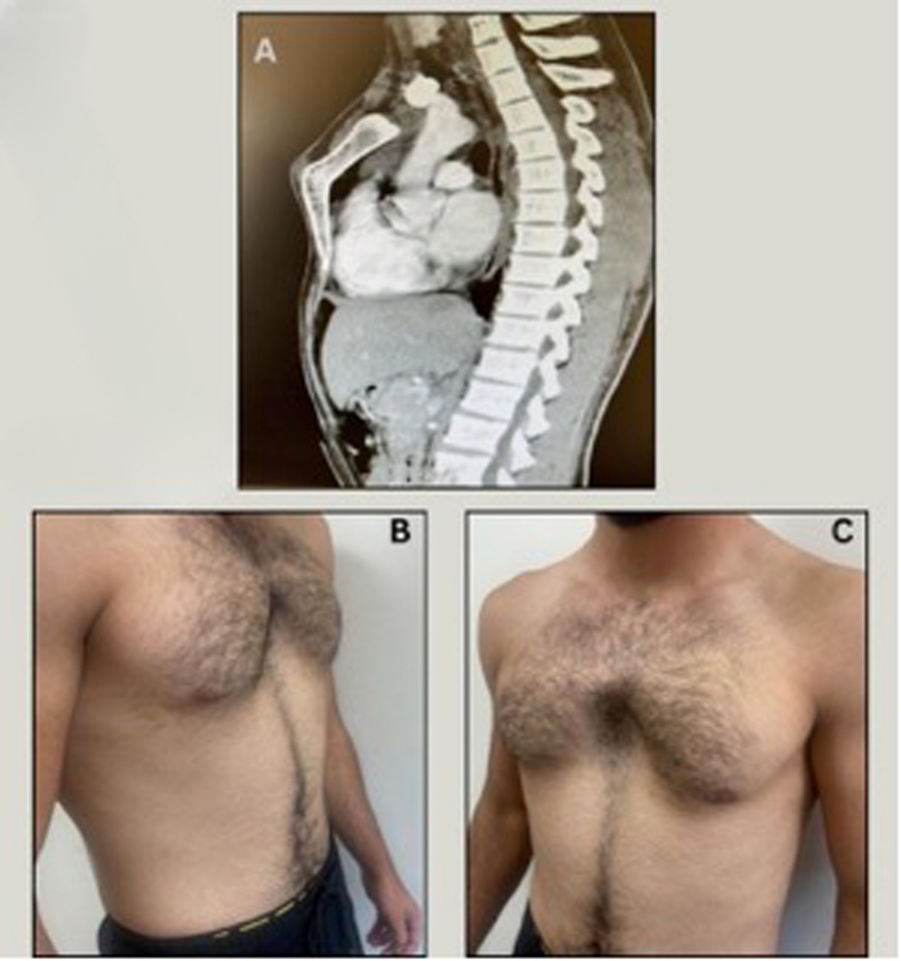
(A) Pre-operative CT lateral view | case 1. (B) Pre-operative lateral view | case 1. (C) Pre-operative anterior view | case 1.
